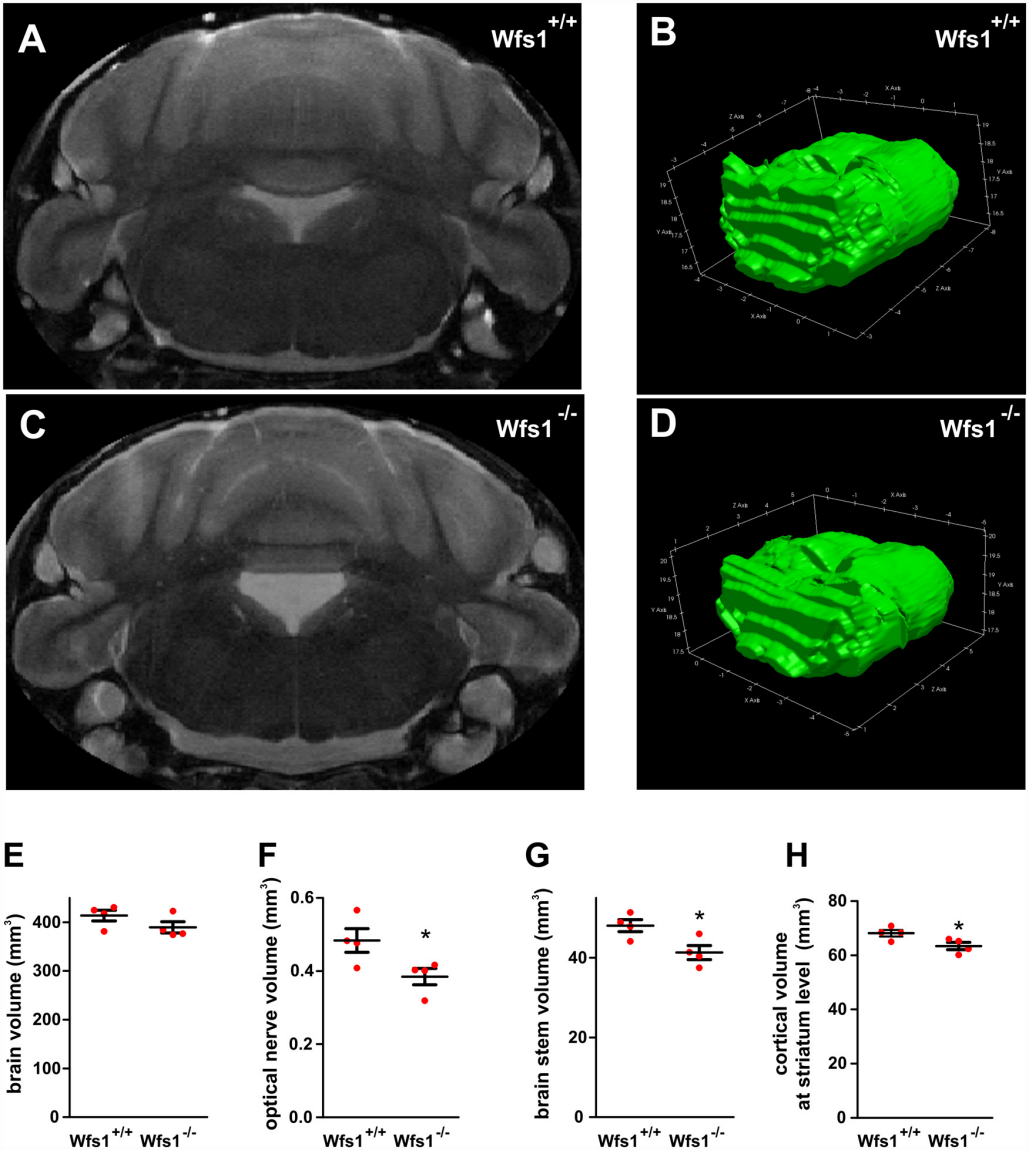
WFS1 deficiency is associated with reduced volume of the optic nerve, brain stem, and cortex at the level of the striatum. (A–D) Brains, within skulls, were scanned *ex vivo* using a 94/20 Bruker BioSpec MRI. Representative single coronal slice from the imaging sequence (A, C) and a 3D reconstruction of the brain stem (B, D). The outer skull, tissue, and surrounding medium have been removed for clarity. (E–H) Volumetric analysis of brains from *Wfs*
